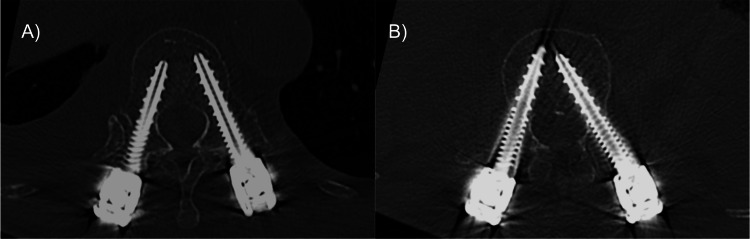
Postoperative CT Confirmation of Pedicle Screw Placement. Postoperative CT images of pedicle screw placement. CT scans showing accurate placement of pedicle screws at the Th12 (A) and L2 (B) levels with no evidence of misplacement.
